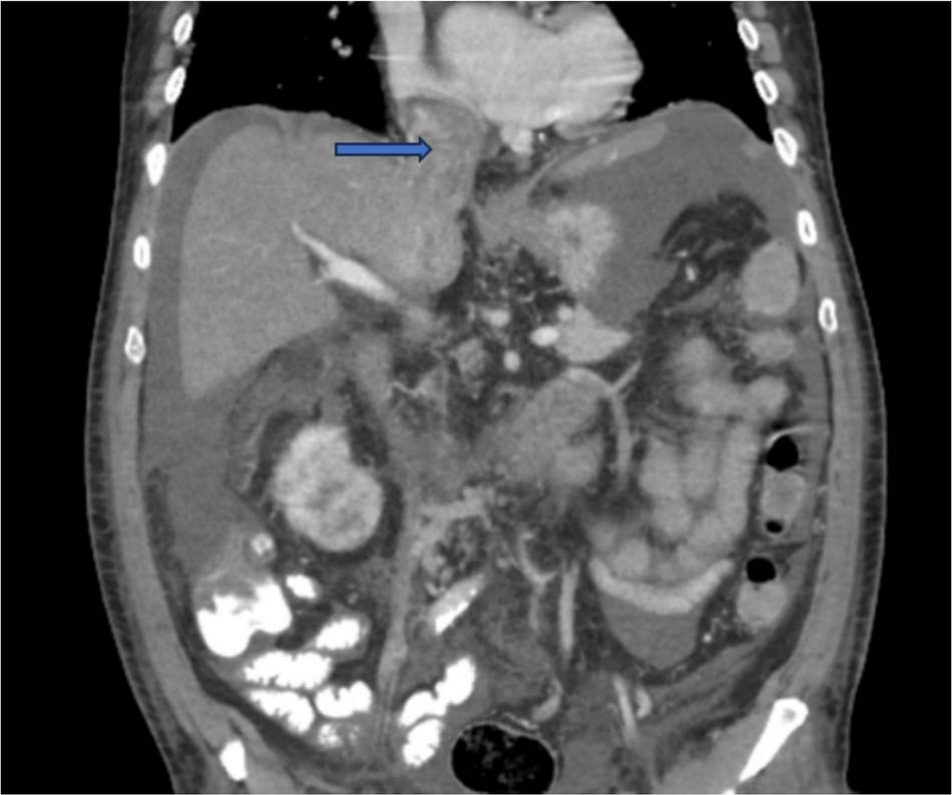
CT chest abdomen and pelvis with contrast showing tumor thrombus is seen within the IVC extending over a long distance from the level of the renal vein cephalad into the right atrium.
